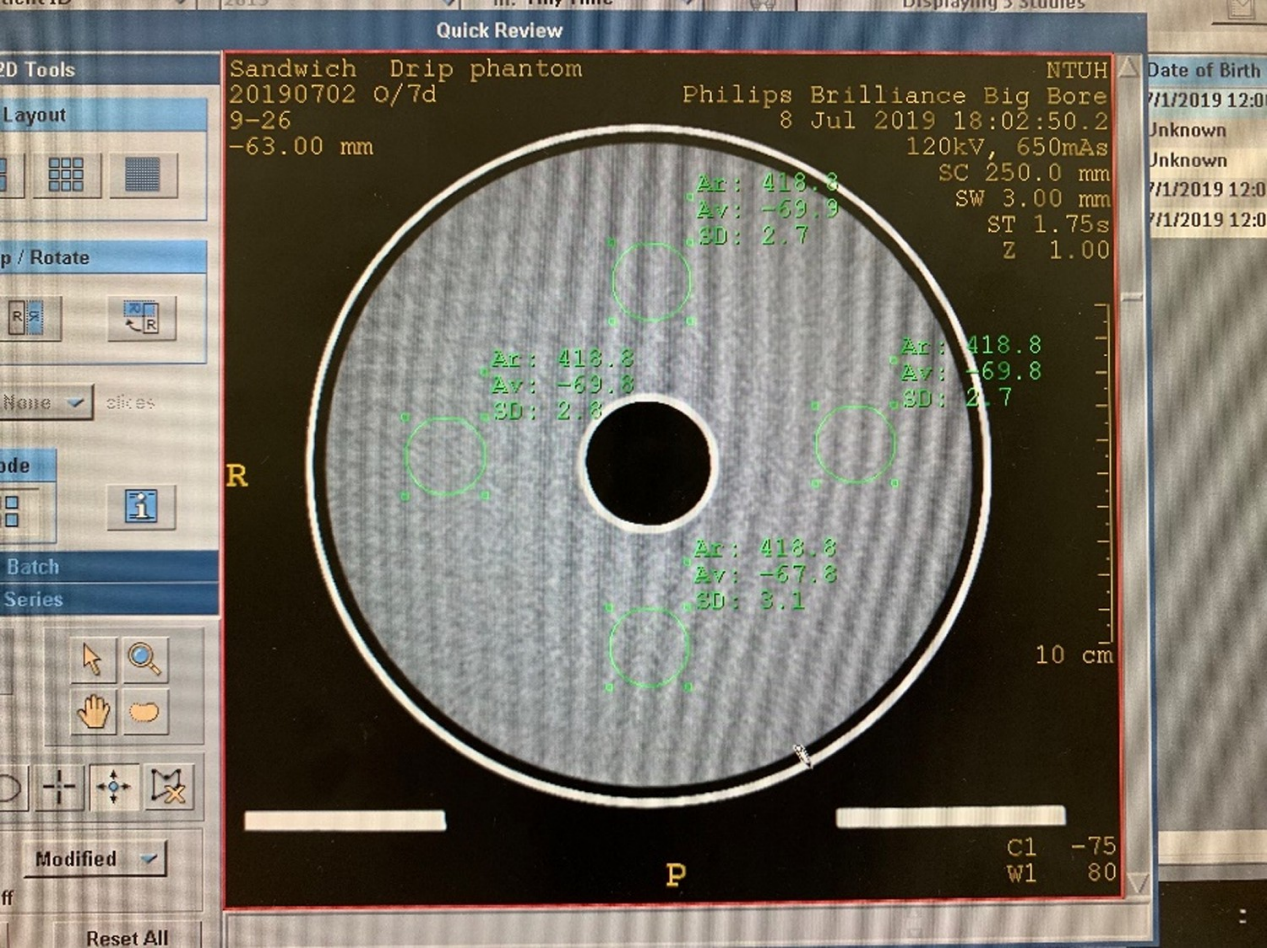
CT image of the wax-filled uniformity slab.
